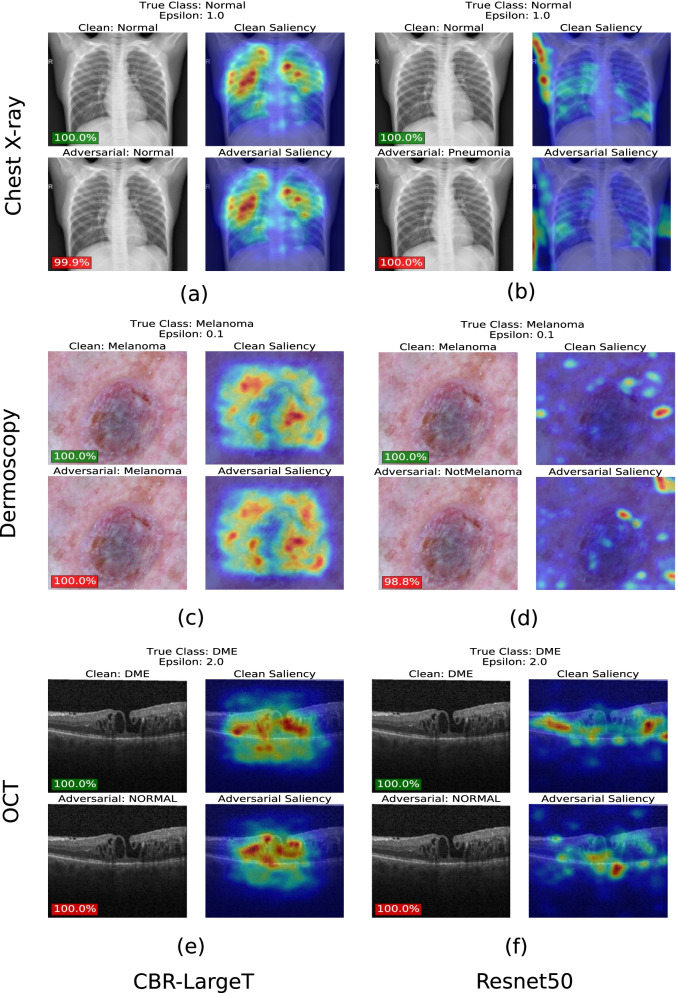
Saliency maps of standard trained CBR-LargeT (a, c, e) and Resnet50 (b, d, & f) for Chest X-ray, Dermoscopy & OCT datasets. Unperturbed images with predicted labels and corresponding saliency maps were visualized on row 1 (no attack) for (a–f), respectively. Imperceptibly perturbed images with predicted labels and corresponding saliency maps were visualized on row 2 (PGD attack) for (a–f), respectively. Saliency maps of CBR-LargeT are more concentrated on the regions of interest whereas Resnet50 includes attention regions that are more sporadic on areas that do not contribute to the classification of the disease
